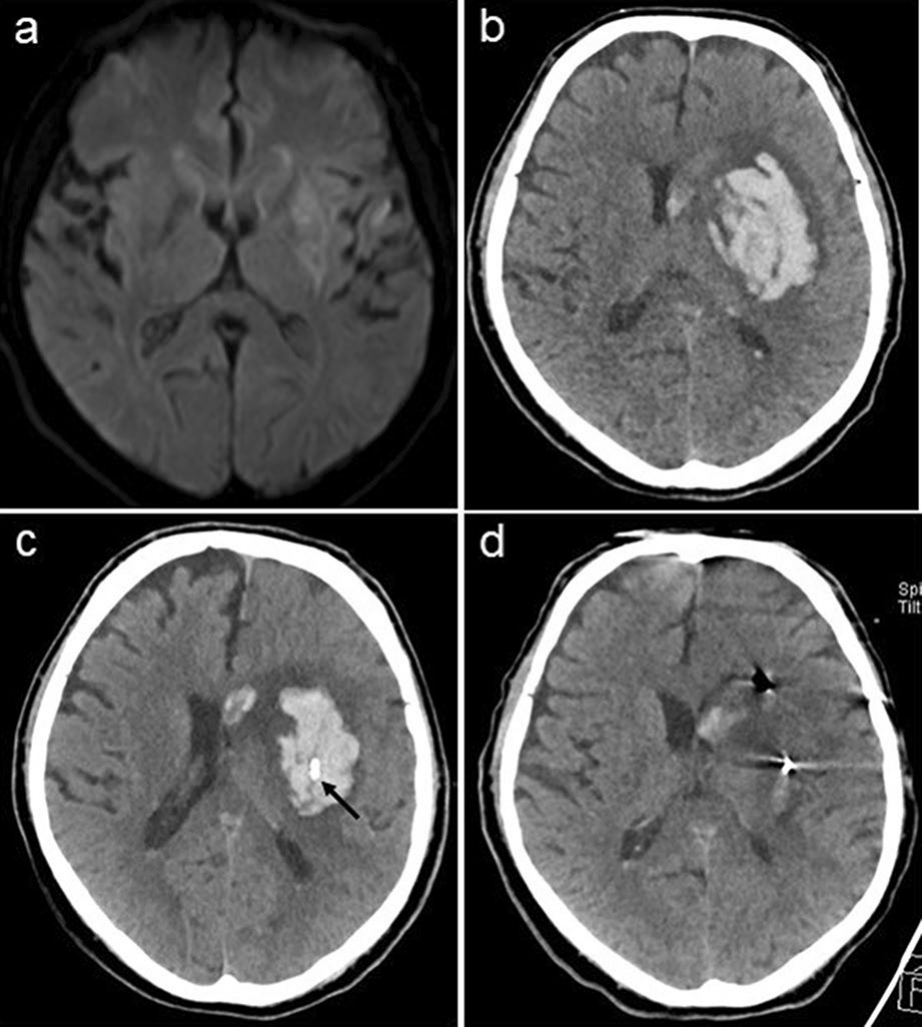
a Magnetic resonance (MR) image of patient 1 at the onset of thrombolysis. b Computed tomography (CT) image of the same patient following clinical deterioration (NIHSS of 25, with somnolence, global aphasia, severe right-sided hemiparesis, dysarthria) 19 h after thrombolysis. Note the large hematoma in the area of the infarct core (59 mL), with diffuse perifocal edema over the left hemisphere (midline shift, 6 mm). c CT image following bedside catheter placement, in which 13 mL of blood was aspirated. The cranial CT image showed good positioning of the catheter (black arrow); however, hematoma size was still substantial (49 mL). The patient’s symptoms had only slightly improved to an NIHSS score of 22; therefore, urokinase administration was initiated. d Cranial CT image showing near complete resolution of the hematoma following urokinase administration (5,000 IU) every 6 h for 24 h
